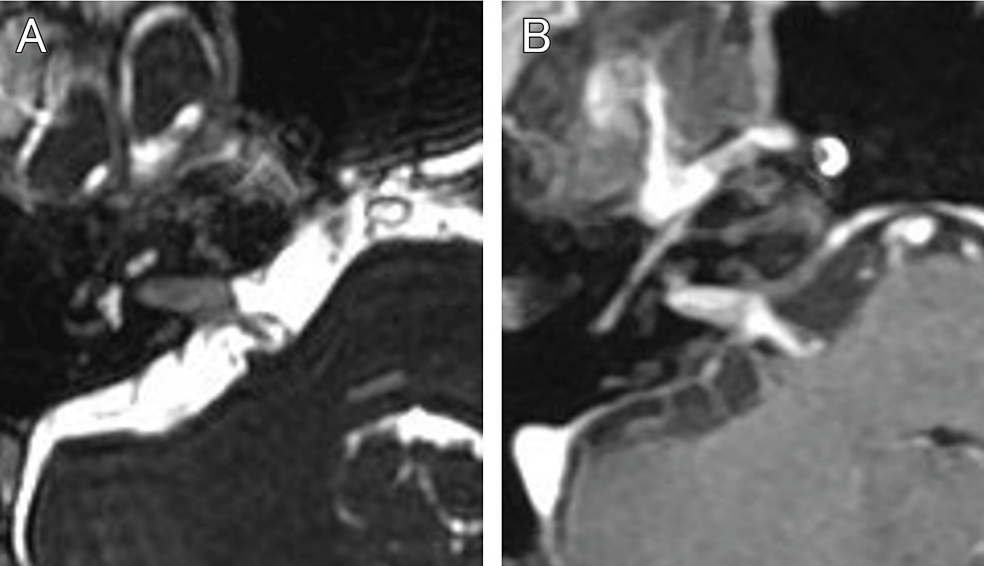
MRI at the initial diagnosis and at the treatment with Gamma Knife radiosurgery The initial T2-weighted image (A) and contrast-enhanced T1 image (B) show a tumor partially protruding from the internal auditory canal into the cisterna. MRI: magnetic resonance imaging
